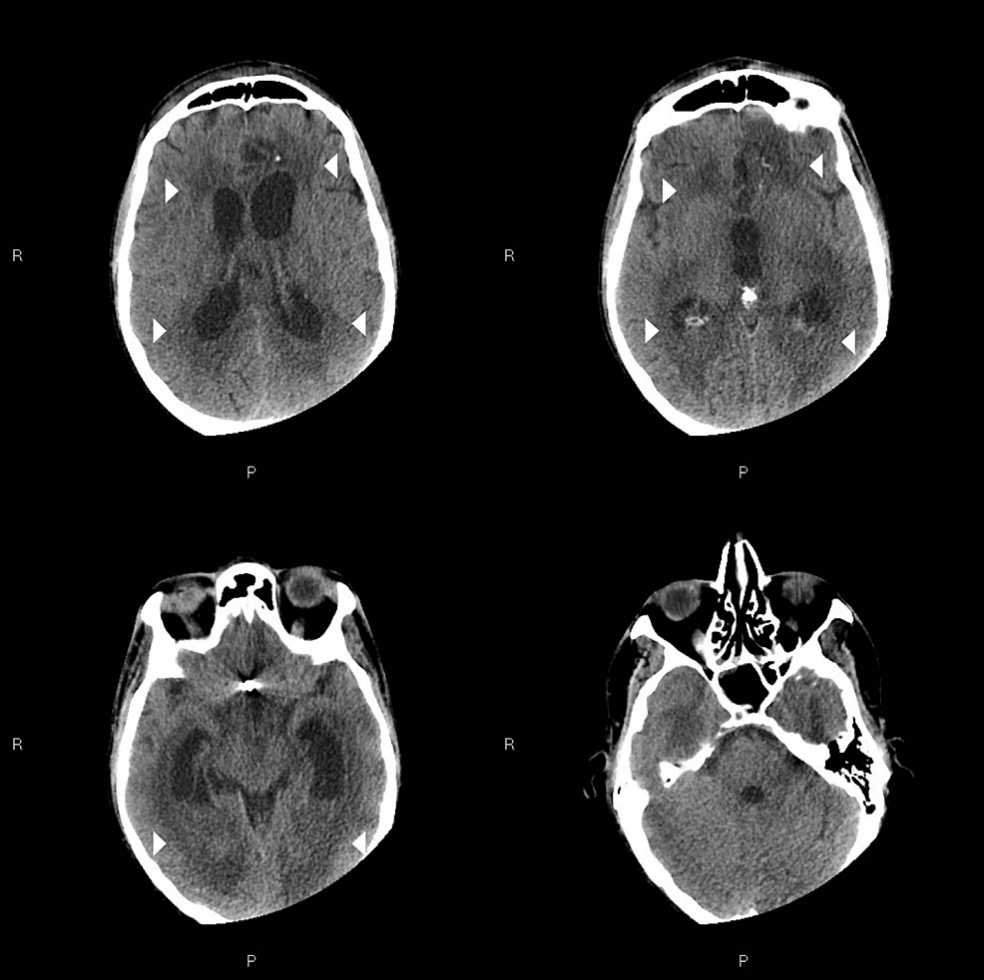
Non-enhanced computed tomography scan demonstrating the onset of acute hydrocephalus Four days after a positive COVID-19 test, neurological status of the patient rapidly worsened. Non-enhanced computed tomography scan demonstrated acute hydrocephalus, mainly involving supratentorial system. Periventricular hypodensity due to the transependymal cerebrospinal fluid resorption is demonstrated (white arrowheads).
